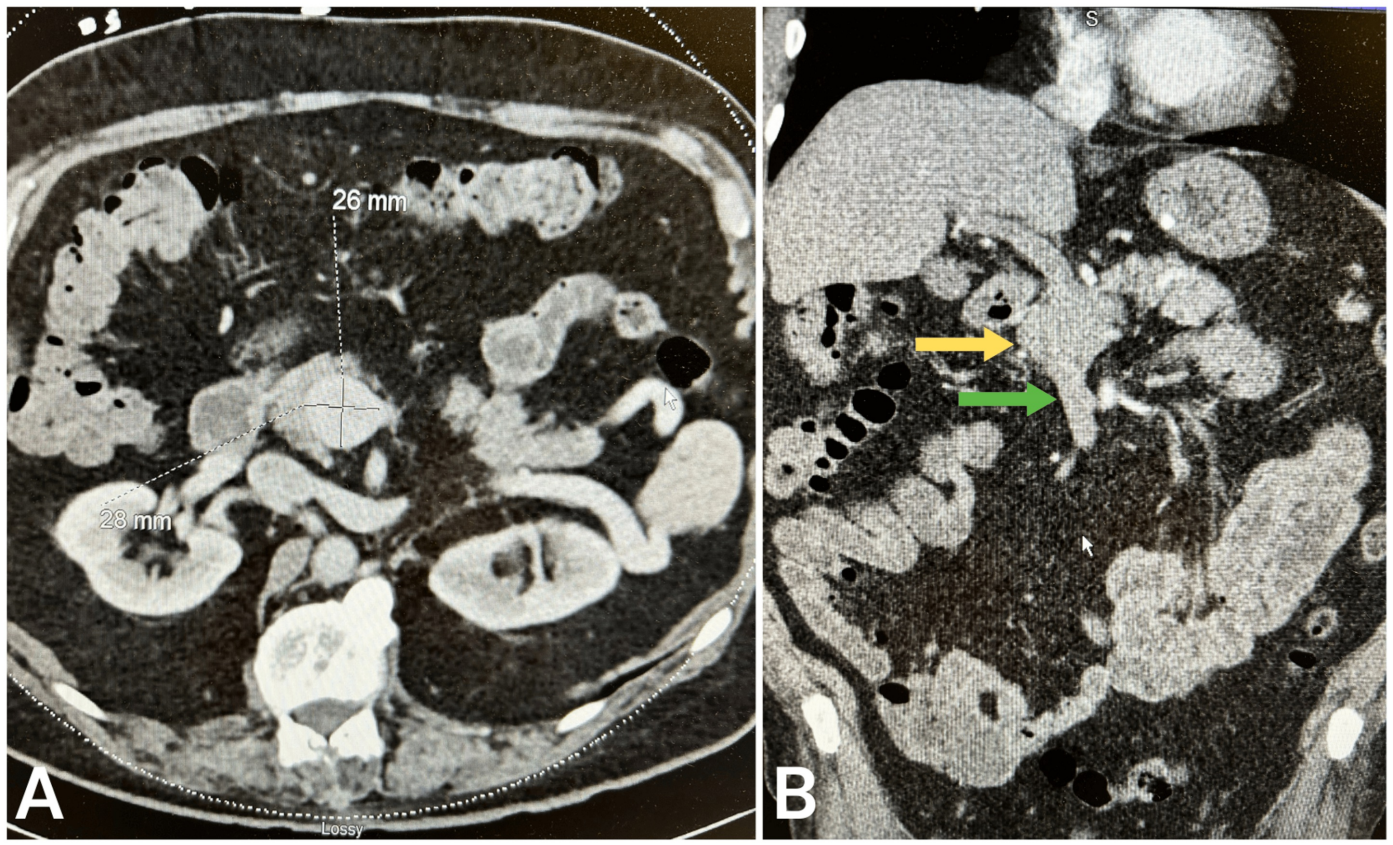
Abdominal computed tomography angiography (CTA) showing a portal vein aneurysm (yellow arrow) above the confluence (green arrow) measuring up to 2.8 x 2.6 x 4.9 cm. (a) Axial view. (b) Coronal view.
